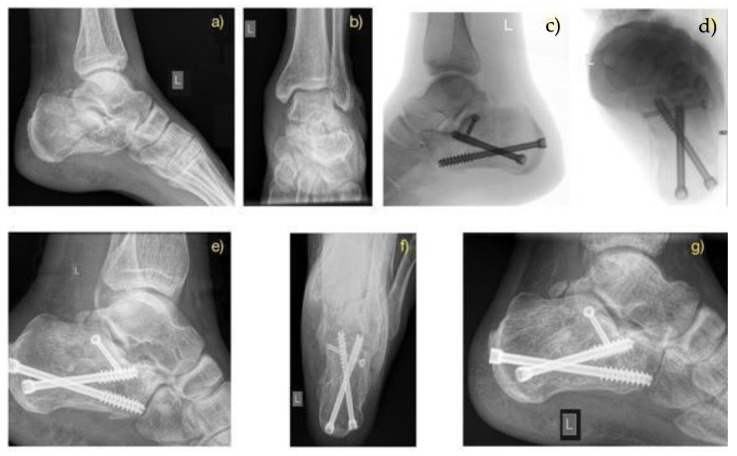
Fracture Case treated with minimal invasive technique according to Fröhlich at al. Preoperative X-ray: lateral (a) and axial (b), Intraoperative pictures (c,d), 14-day postoperative X-ray of left hindfoot (e,f). Follow-up after 12 months (g).
